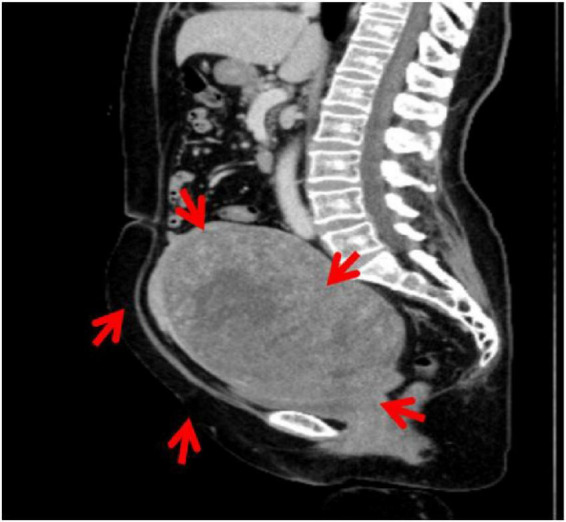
Enhanced abdominopelvic CT of Case 1: A round-like soft-tissue mass measuring 17.8 cm × 15.1 cm × 12.3 cm is seen in the abdominal cavity with heterogeneous density, showing multiple tortuous thin vascular signals and non-enhanced liquefactive necrotic areas.
